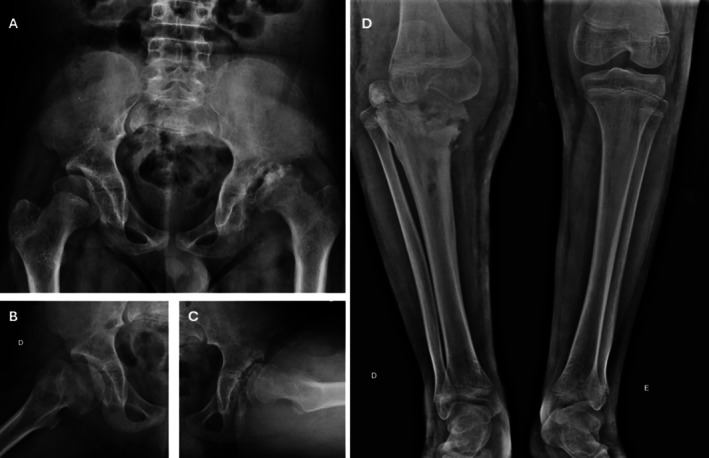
Pelvic and lower‐limb radiographs. The acetabula have a steep and shallow contour. The femoral heads have lost their normal rounded shape, with reduced centre‐edge angle and shallow acetabulum, consistent with acetabular dysplasia, accompanied by deformity and degenerative changes of the femoral heads, mainly on the left side (A–C). The right knee shows degenerative changes, including joint space narrowing, osteophyte formation, and subluxation (D).
